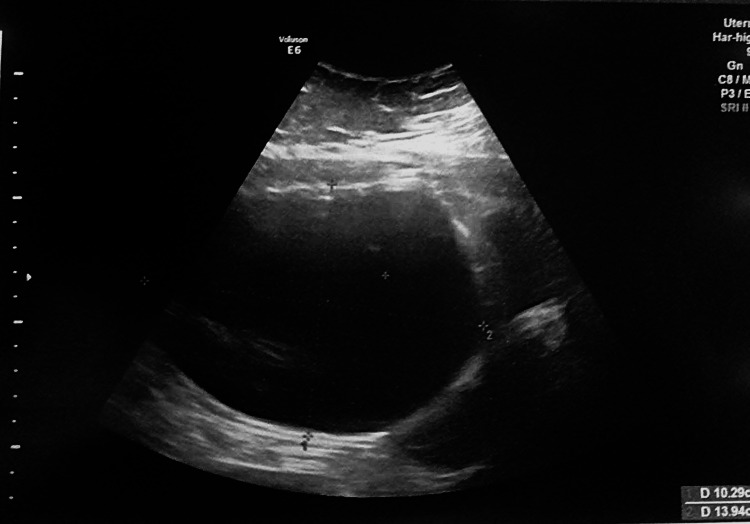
Ultrasound imaging showing a fluid filled massive cyst.
